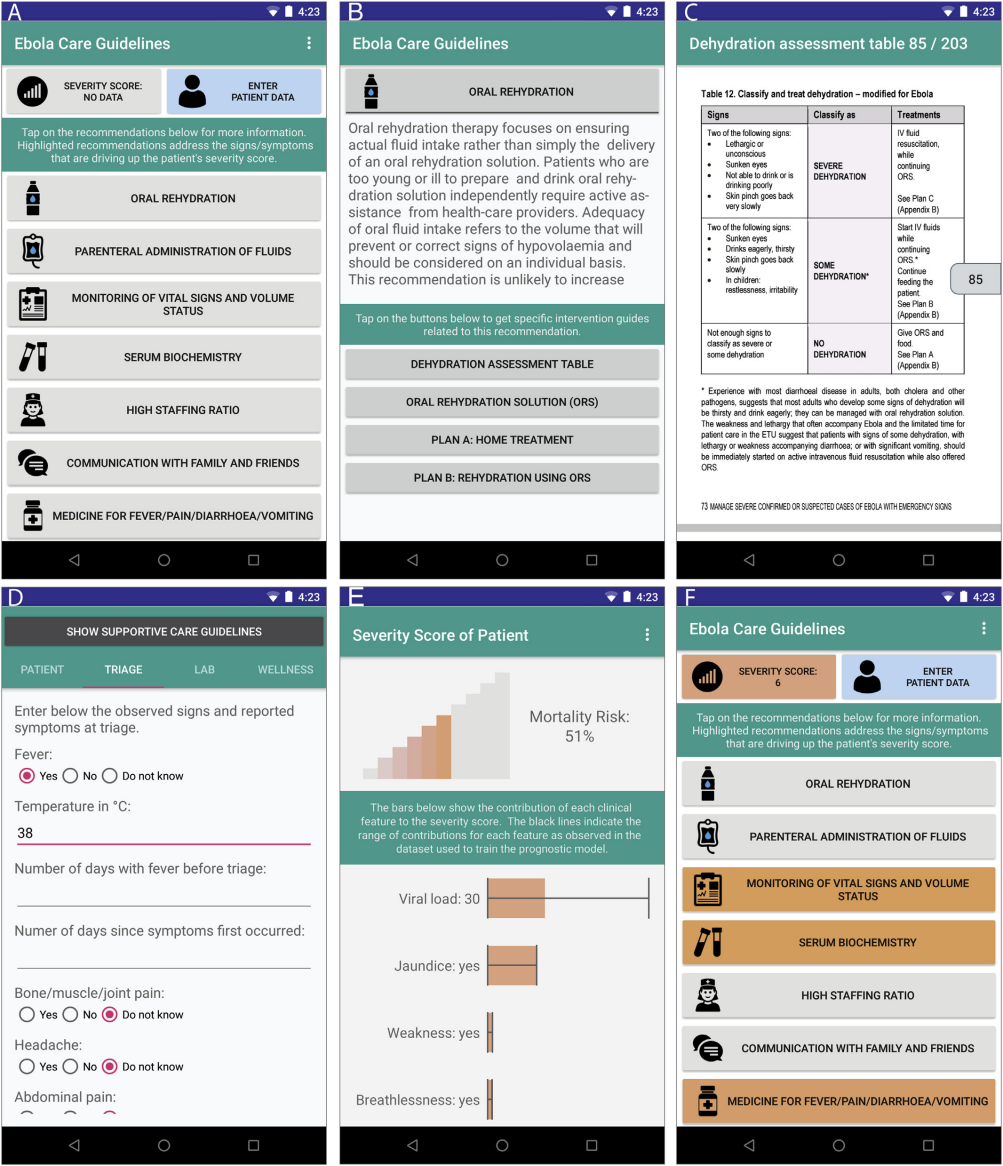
Ebola Care Guidelines app. The home screen presents the list of recommendations (A), which can be selected to access specific interventions associated with each recommendation (B). Selecting a specific intervention or guideline redirects the user to the corresponding section in the WHO's manuals for care and management of hemorrhagic fever patients (C). The app allows the users to enter basic demographic information (age, weight), vitals, signs & symptoms at presentation, lab data (CT value from first RT-PCR and malaria test result), and wellness scale (D). Based on the available data, the app calculates the severity score of the patient using the suitable prognostic model and presents a customized risk visualization (E). The recommendations that are associated with the presentation signs and symptoms are highlighted in the home screen (E).
